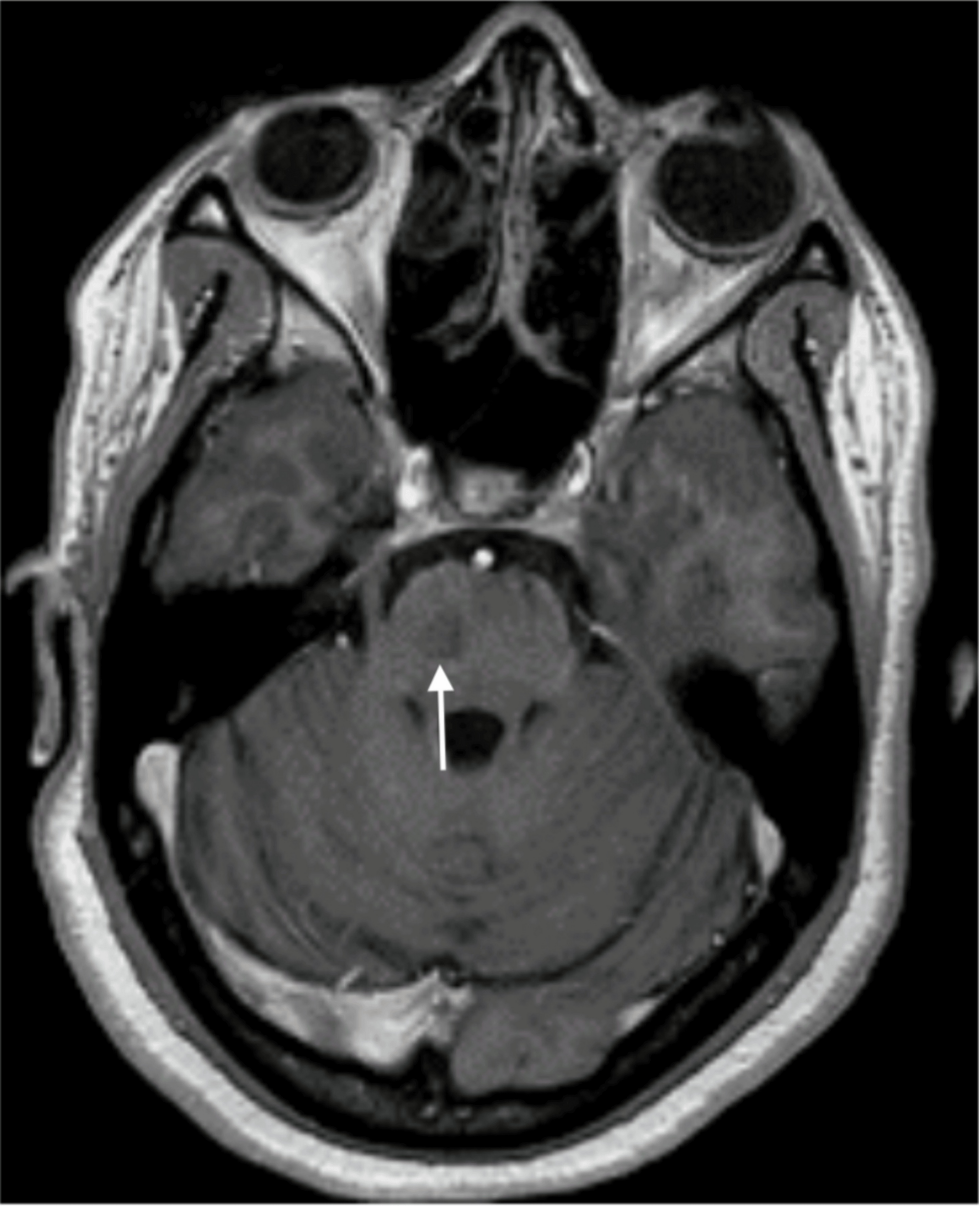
Postictal brain MRI centered on the pons (axial section, T1-weighted enhanced imaging) The same right lateral pontine lesion, as seen in Figures 3, 4, shows no enhancement post-gadolinium injection (white arrow) MRI: magnetic resonance imaging
